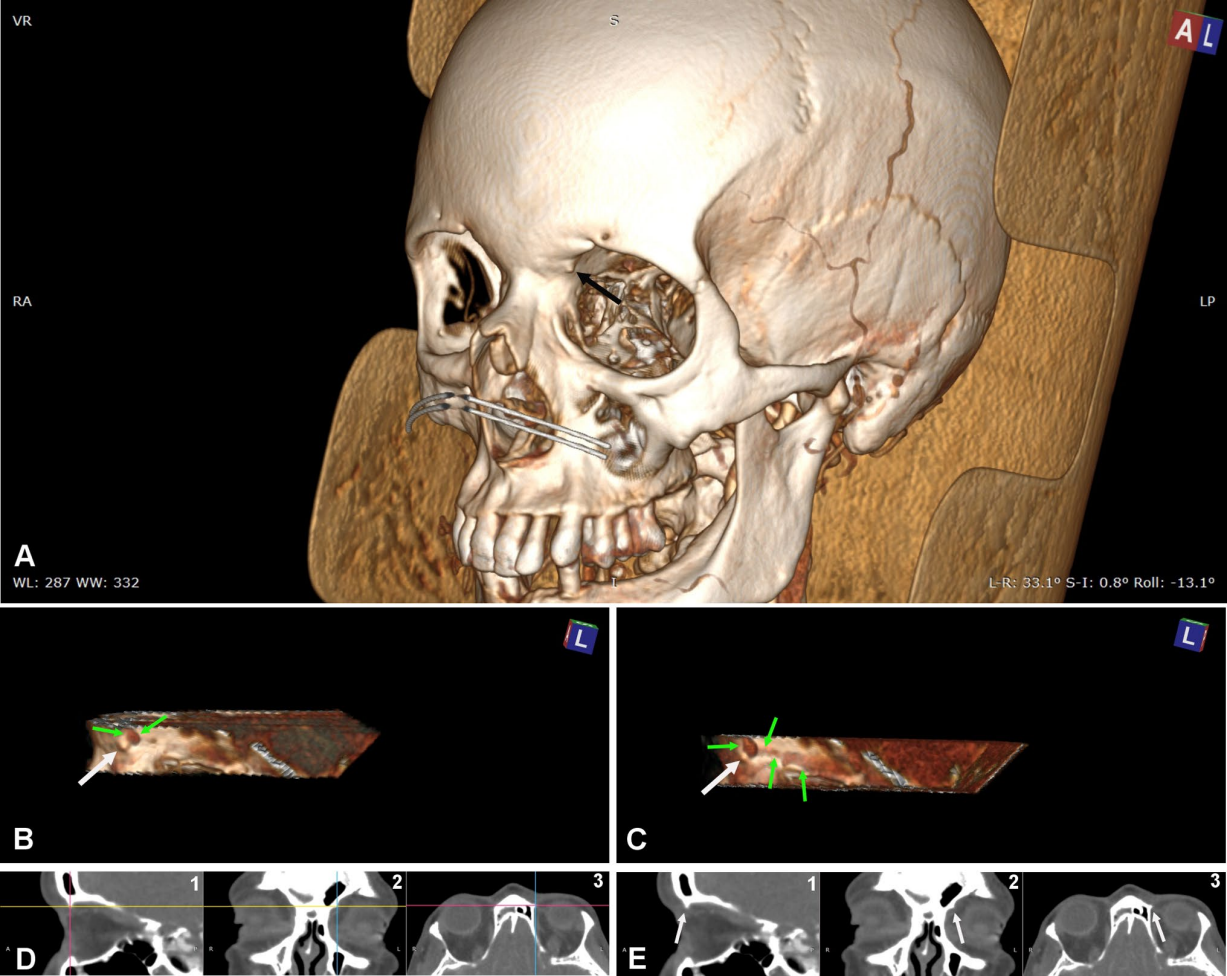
CT of a left orbit with a trochlear tubercle (TT_2_). The location of TT_2_ appears different from TT_1_. A A TT_2_ can be seen within the orbit (arrow). B, C Lateral view of the orbit with the lateral wall and the orbit content clipped away for a better visualization of the medial wall with associated tubercle (white arrow). The adjustment of the image window in C allows to see the transversely cut reflected part of the tendon (green arrows) located over and behind TT_2_. A different adjustment of the image window in D allows to see the shade of the superior oblique muscle and tendon that courses over and behind TT_2_. D, E The same TT_2_ can be seen in MRP. D demonstrate the orientation of the planes intersecting TT_2_. D1 shows the orientation of the frontal (magenta) and axial (yellow) planes as seen on the sagittal plane; D2 shows the orientation of the sagittal (blue) and axial (yellow) planes as seen on the frontal plane; D3 shows the orientation of the frontal (magenta) and sagittal (blue) planes on the axial plane. E is the same image seen in D without showing the planes to better visualize TT_2_ (white arrows) on the sagittal (E1), the frontal (E2) and the axial (E3) planes
